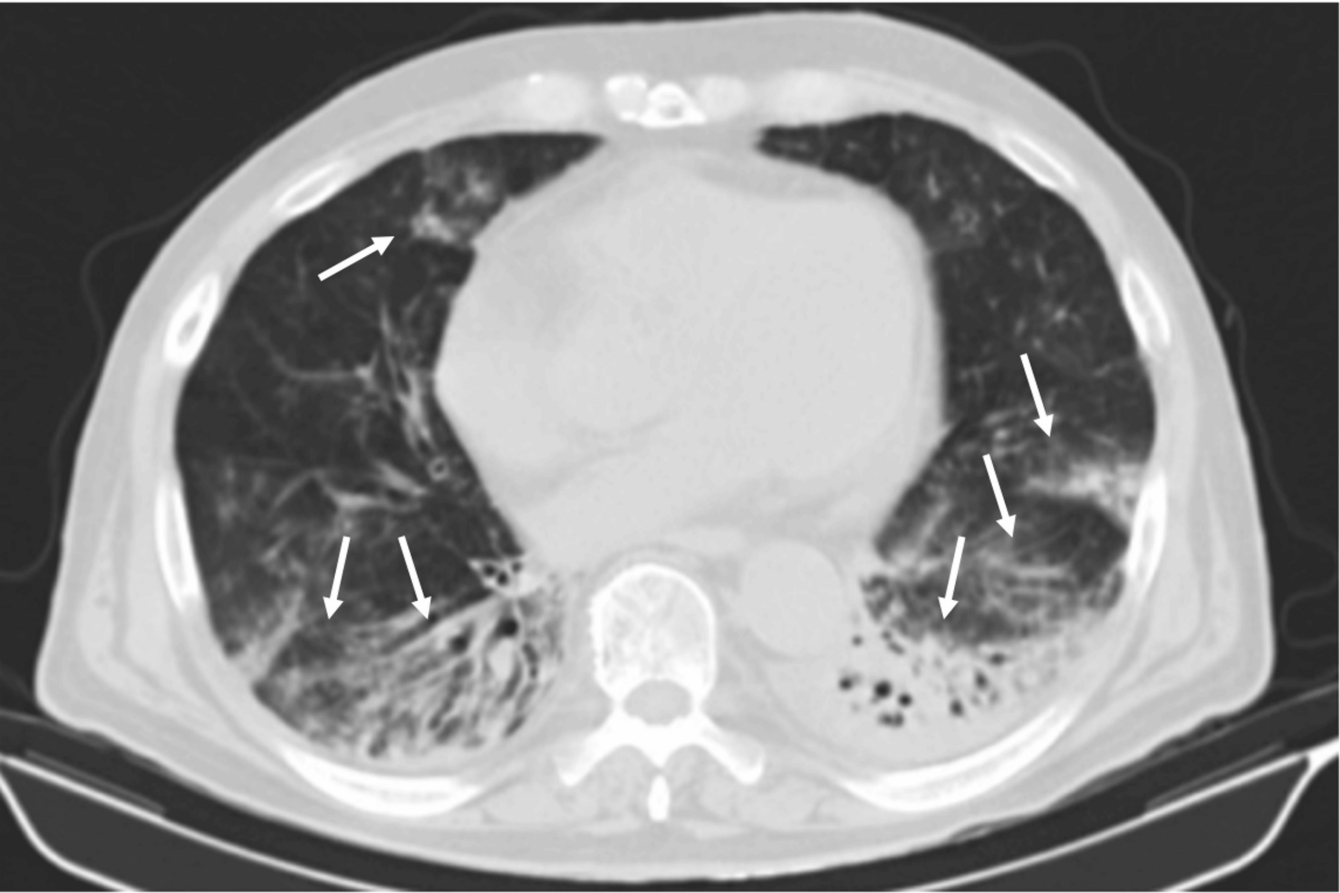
Chest CT scan with bilateral pneumonia of VZV infection showing multiple bilateral pulmonary consolidations (arrows) CT: computed tomography, VZV: varicella-zoster virus
